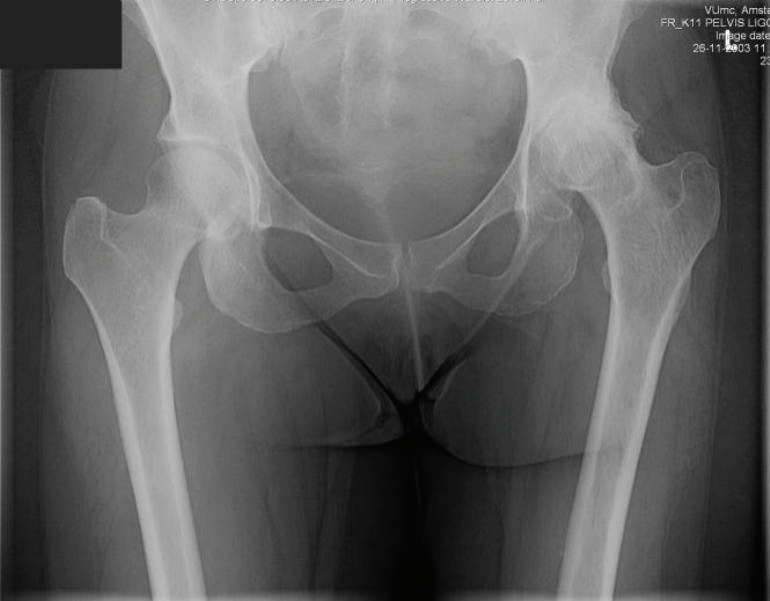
Radiograph of the pelvis shows ankylosis of the left hip joint. The femoral head filled up the acetabular defect.
